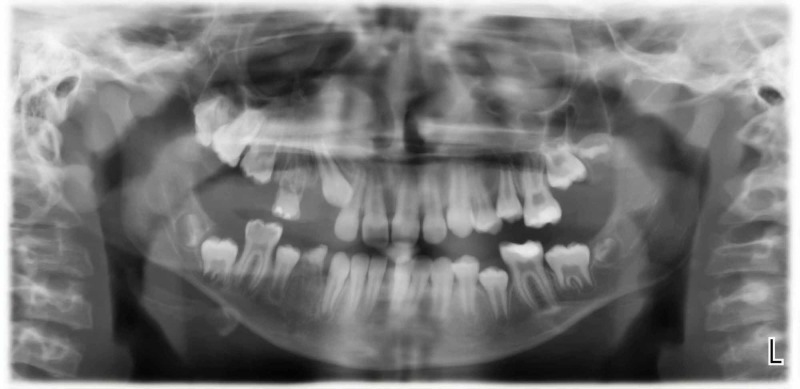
A dental radiograph demonstrating hyperplasia of the right maxillary alveolus and basal bone with missing teeth.
